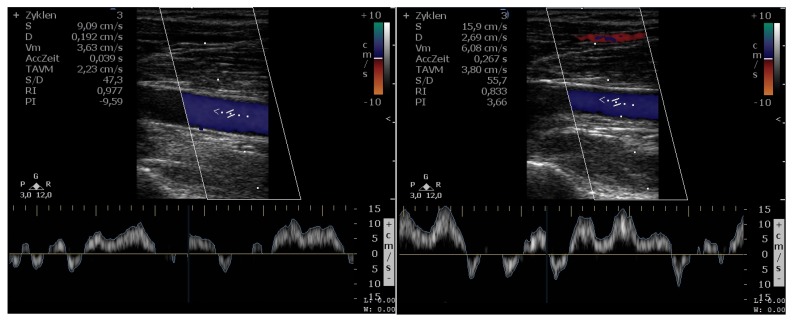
Duplex ultrasonography of the vein. Left: ratio of the stimulation duration to the beat-to-beat interval of 40%; right: ratio of the stimulation duration to the beat-to-beat interval of 56%.
